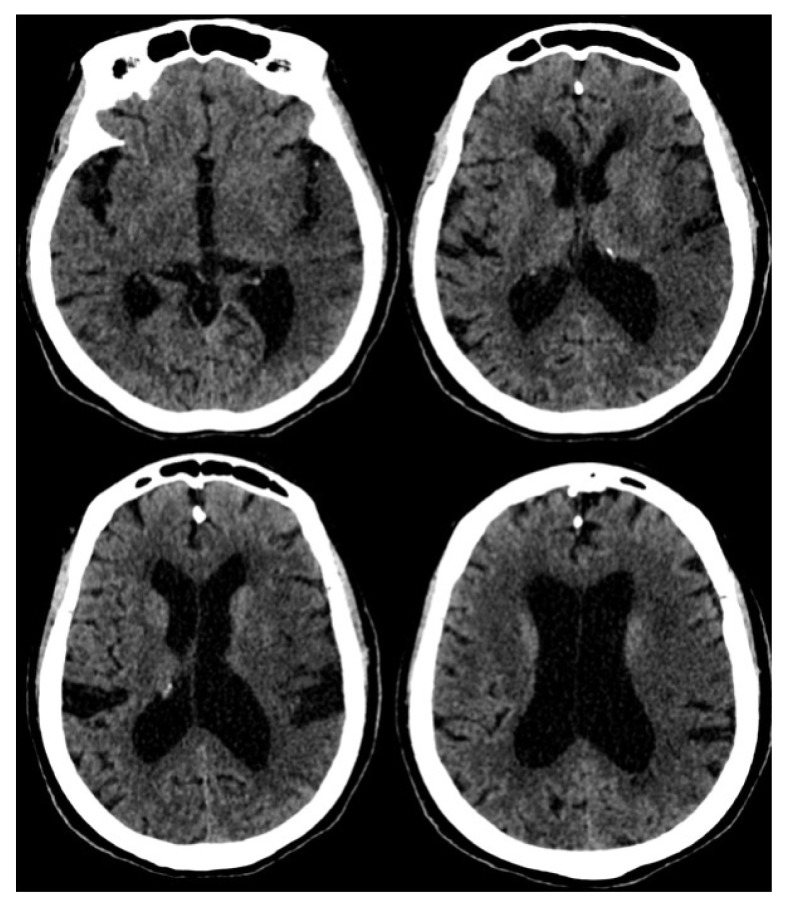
Non-contrast CT brain of Madam M presenting with right hemiparesis. The image shows an ill-defined hypodensity in the left middle cerebral artery territory involving segments M2 to M6 and the left insular cortex. There is loss of grey–white matter differentiation, effacement of sulci, and loss of the insular ribbon sign. No evidence of acute intracranial haemorrhage is seen
